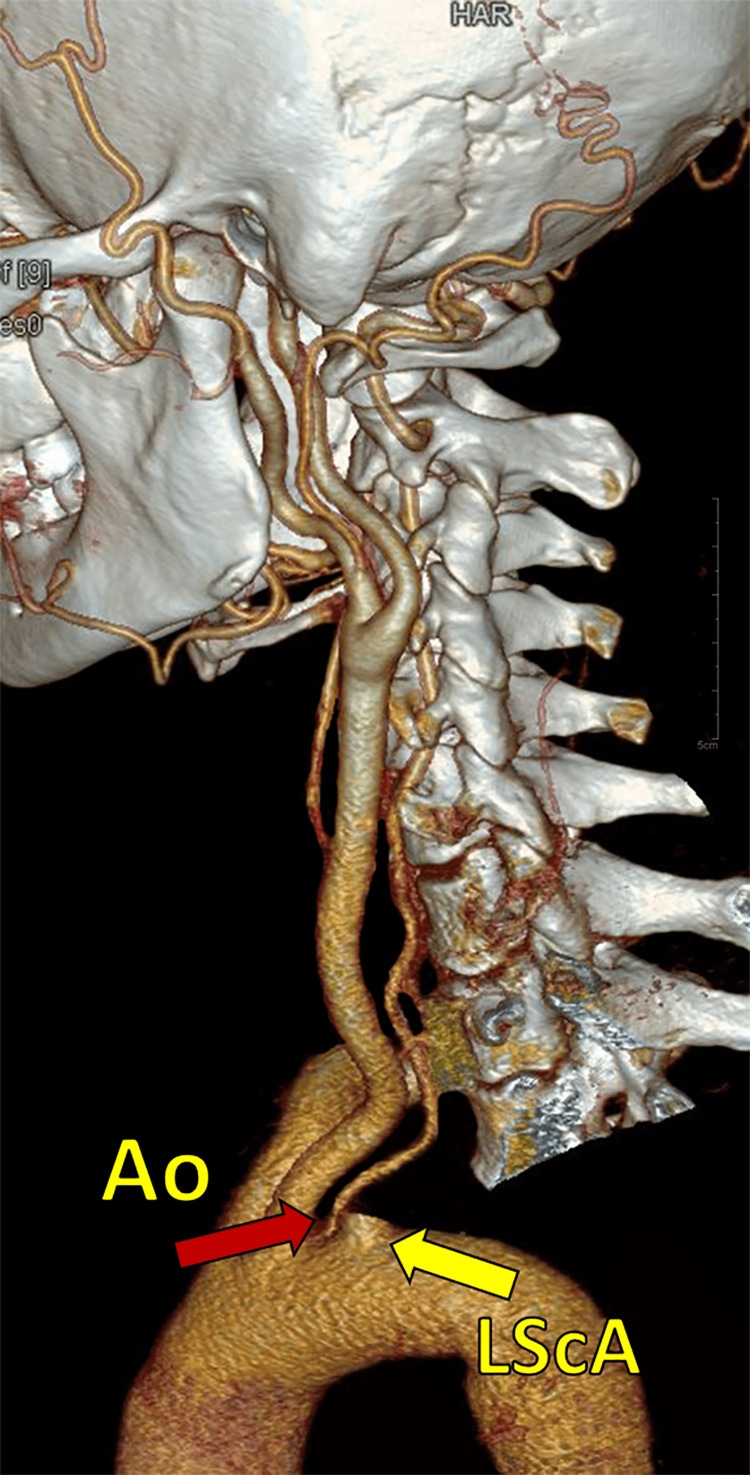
CT angiography 3D reconstruction image showing aortic origin (red arrow, Ao) of the left vertebral artery. The origin from the left subclavian artery has been cut in the image (yellow arrow, LScA) for better visualization of the aortic origin.
